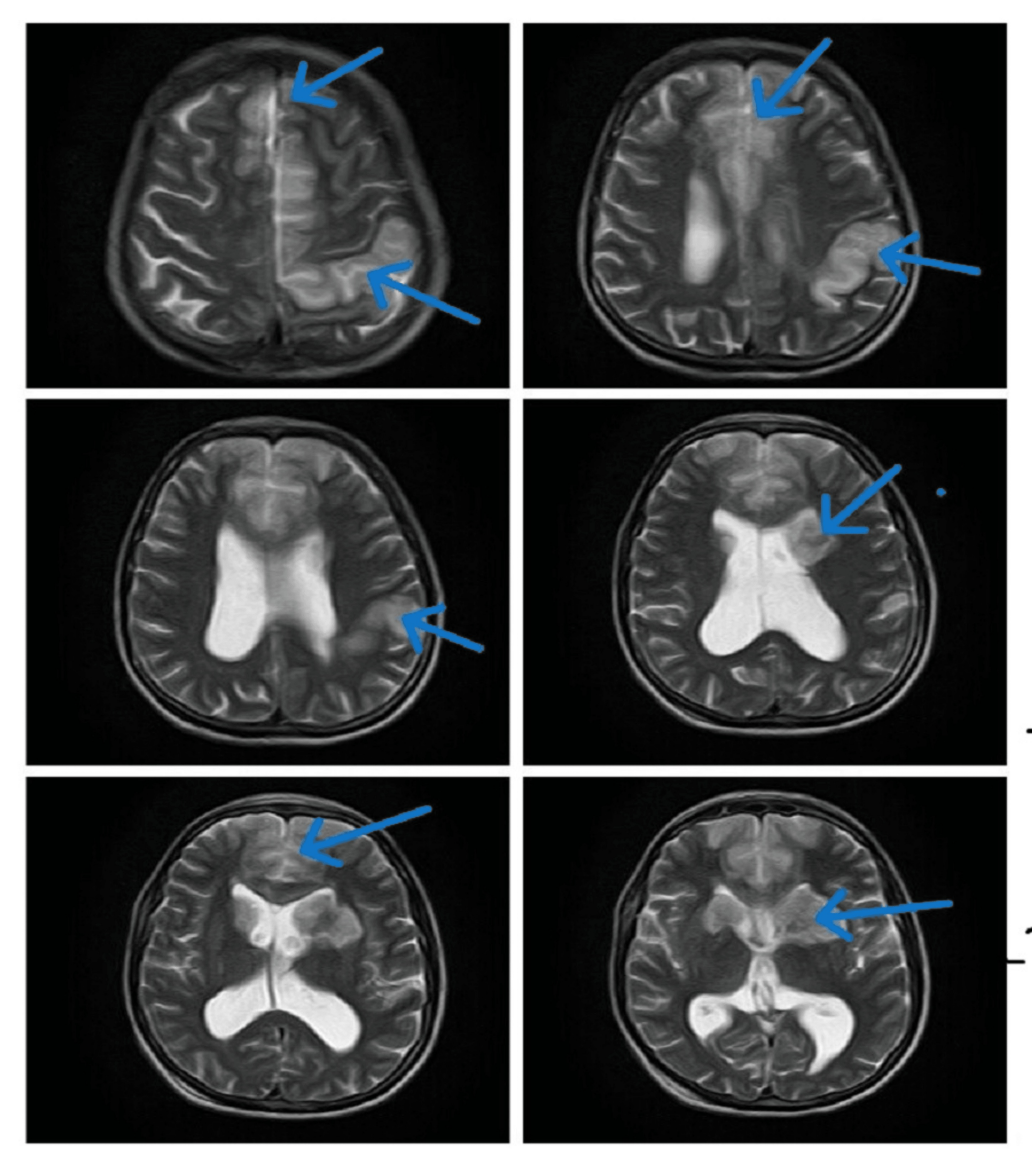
T2-weighted imaging (T2WI) MRI (axial view) shows multifocal ischemia (blue arrows)
